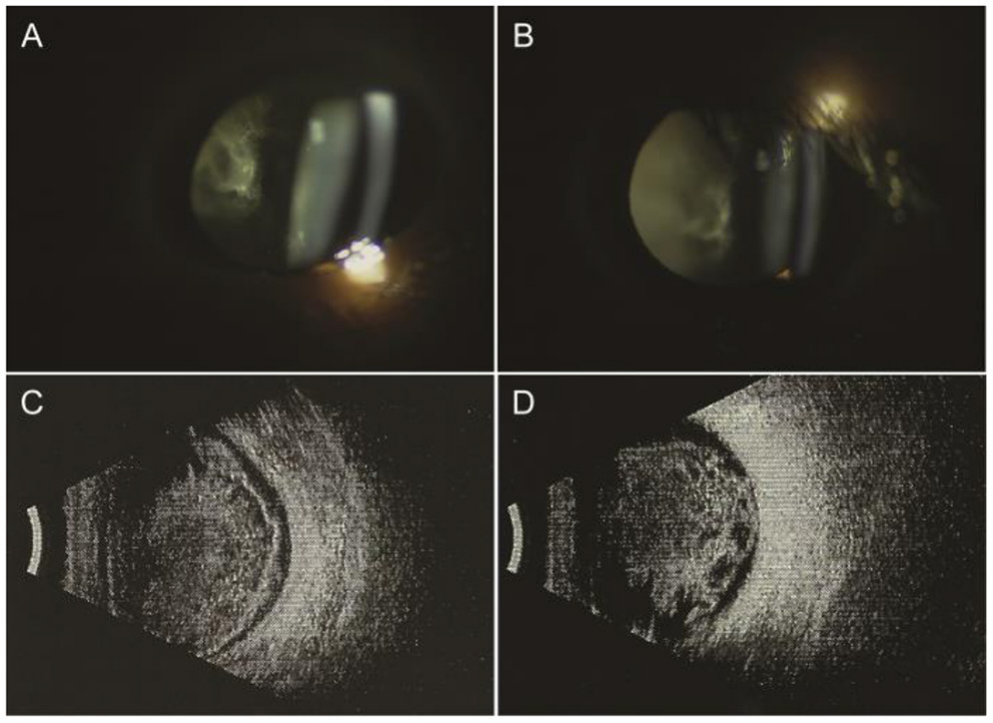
Ophthalmic examination before the first operation. Anterior segment examination showed white vitreous opacities (footplates) adhering to the posterior lens capsule. B-scan ultrasonography revealed that the glass-wool vitreous opacities were almost completely detached except for the local adhesion to the retina and optic disc. (A,C) Right eye; (B,D) left eye.
